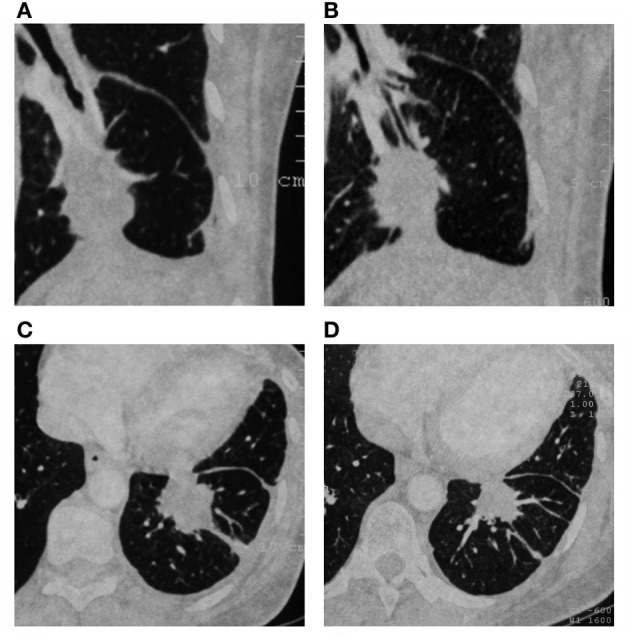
Stable disease after maintenance treatment with Racotumomab and pemetrexed. (A,C) Computed tomography in March 2010 before treatment. (B,D) Computed tomography in February 2012, last assessment.
